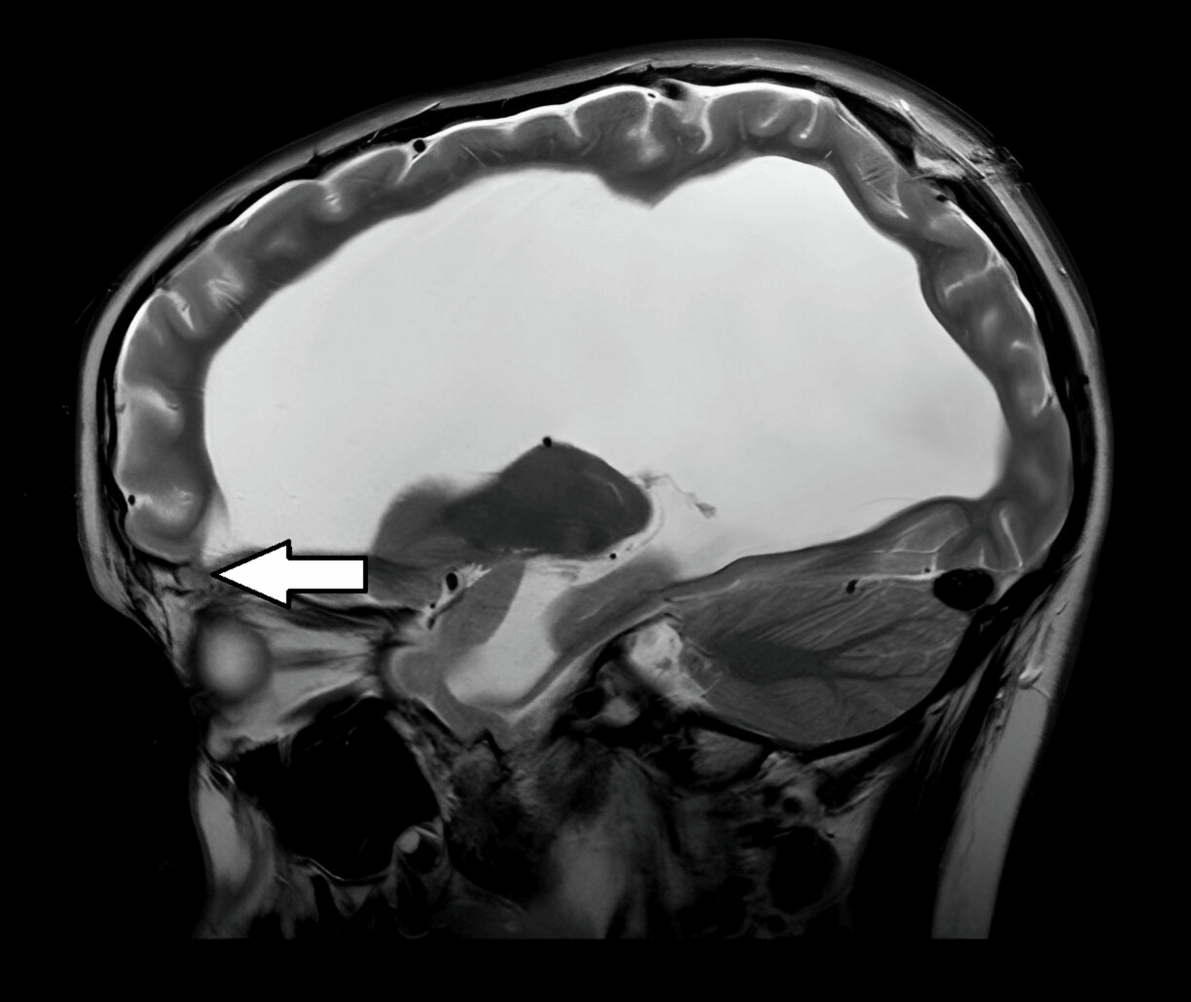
Postoperative magnetic resonance imaging findings (arrow pointing to the herniated meninges through the orbital roof defect)
